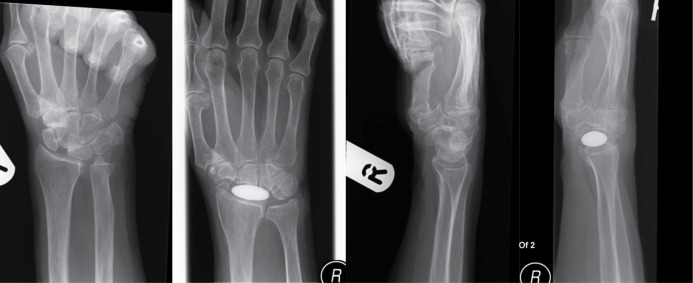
Post-operative radiographs of a patient with an Amandys® for osteoarthritis of the wrist and scapholunate advanced collapse
